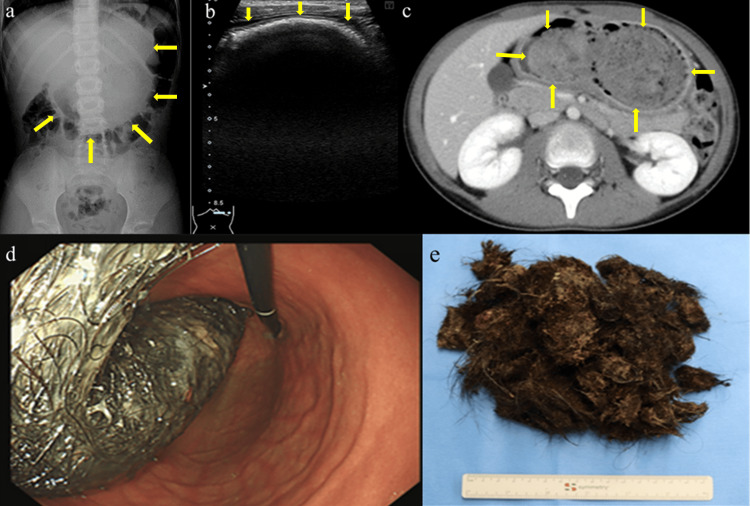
Imaging and macroscopic findings of the trichobezoar (a) An abdominal radiograph shows a mass with a distended stomach and displaced transverse colon. (b) An abdominal ultrasound scan shows an arc-like hyperechoic curvilinear casting with a clear posterior acoustic shadow. (c) Axial contrast-enhanced CT scan showing a well-defined, heterogeneous, and non-enhanced mass. (d) The endoscopic image shows a large, hard, black hairball occupying the entire lumen of the stomach from the fundus to the pylorus. (e) The trichobezoar (201 g) that was removed from the stomach CT: computed tomography
